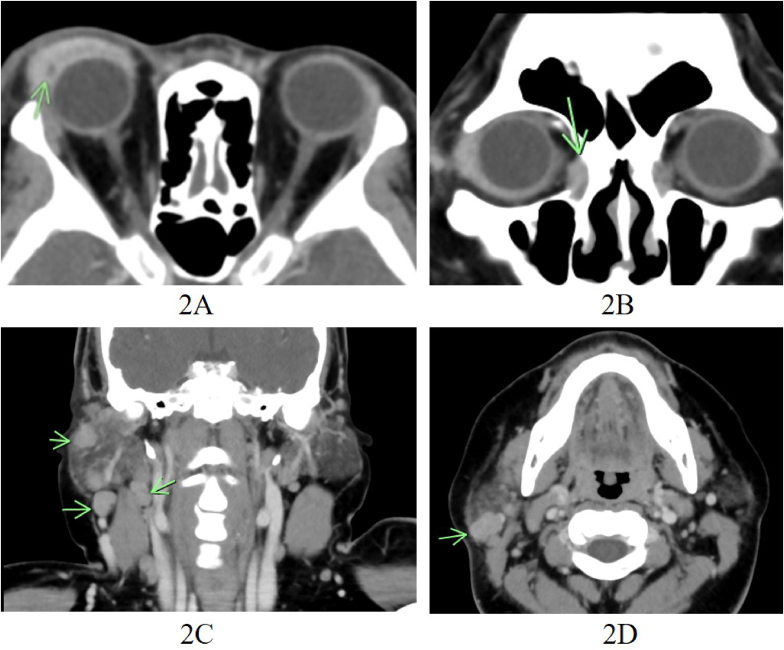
Contrast-enhanced computed tomography scan 2A. An ill-defined enhancing soft tissue density lesion involving the right upper eyelid, with a rim-enhancing lesion inside, compatible with infection with an abscess 2B. The ill-defined soft tissue enhancing lesion at anteromedial aspect of the right orbit extending to superior aspect of the right nasolacrimal duct, probable another infectious site 2C and 2D. Multiple enlarged and subcentimeter lymph nodes at right cervical region (2C) and right intraparotid region (2D).
